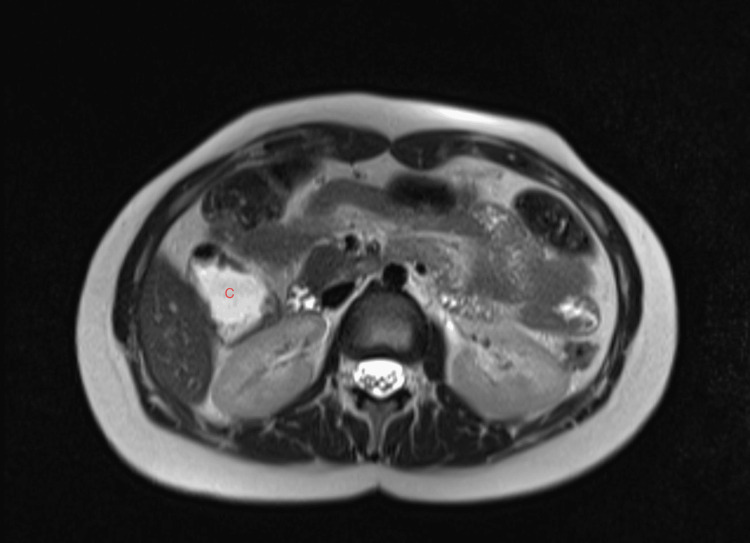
Axial T2-weighted MRI Axial T2-weighted MRI demonstrating absence of the gallbladder, with bowel loops occupying the expected gallbladder fossa (C = colon). MRI: magnetic resonance imaging
